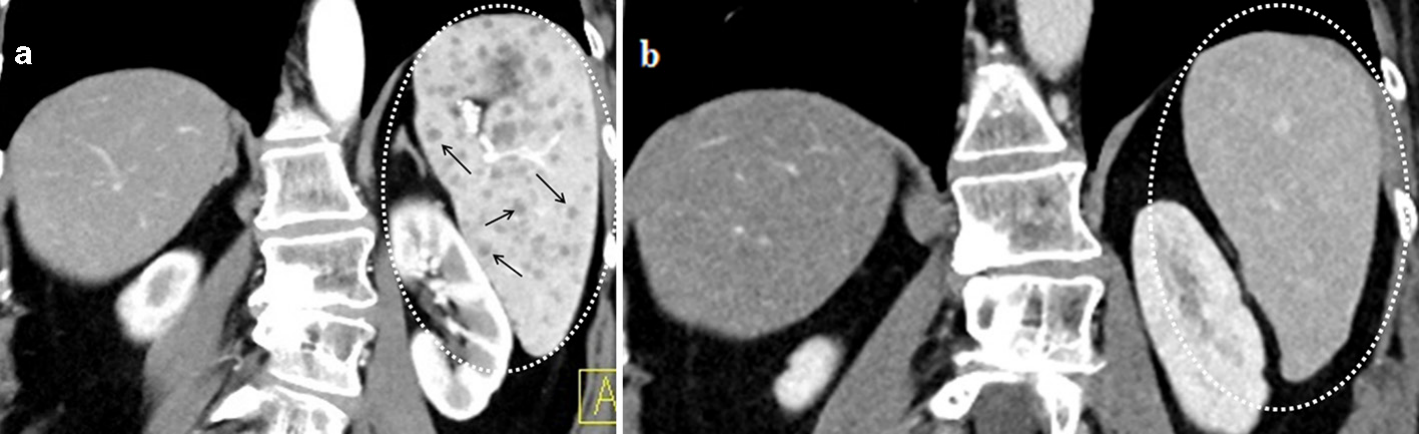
(a) Abdominal contrast-enhanced CT scan showed unnumbered subcentimetric
